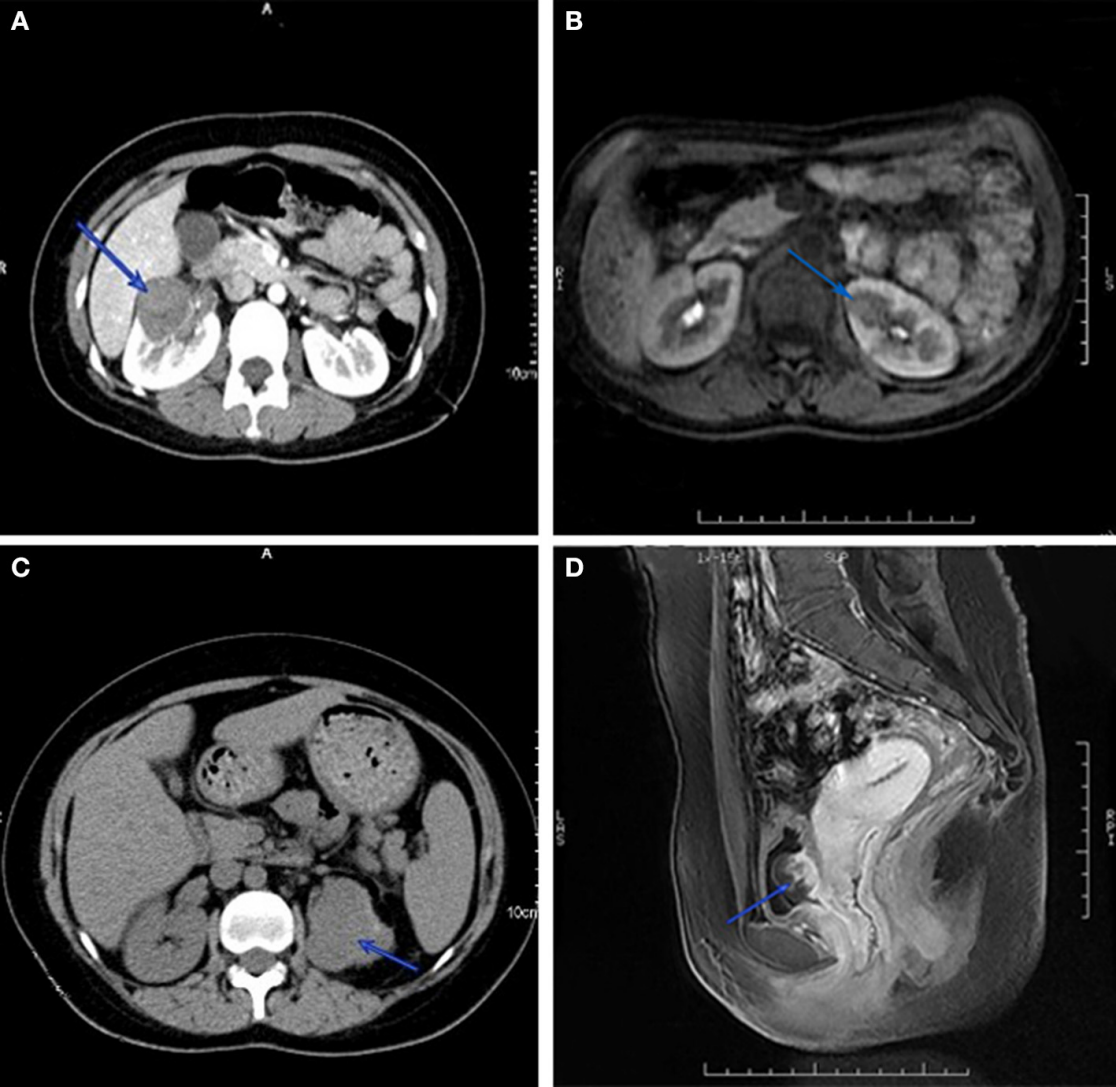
Computed tomography imaging of GTN patients with renal metastasis. Isolated renal mass in right (A) and left kidney (B), and multiple lesions disform the left kidney (C). Magnetic resonance imaging of metastatic lesion in bladder (D).
